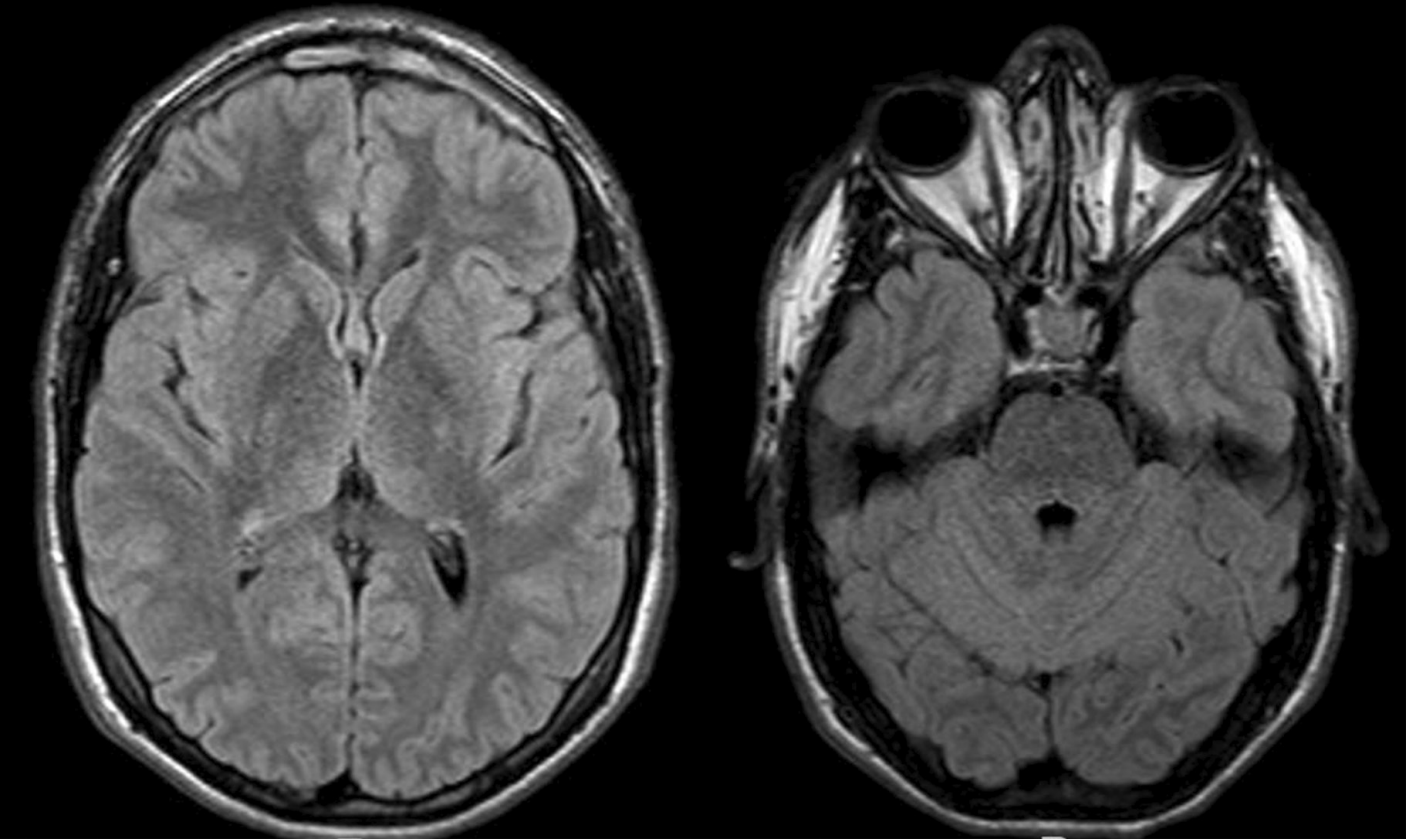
Normal Brain Magnetic Resonance Imaging of the same patient, with no vasogenic edema or sequelae.
